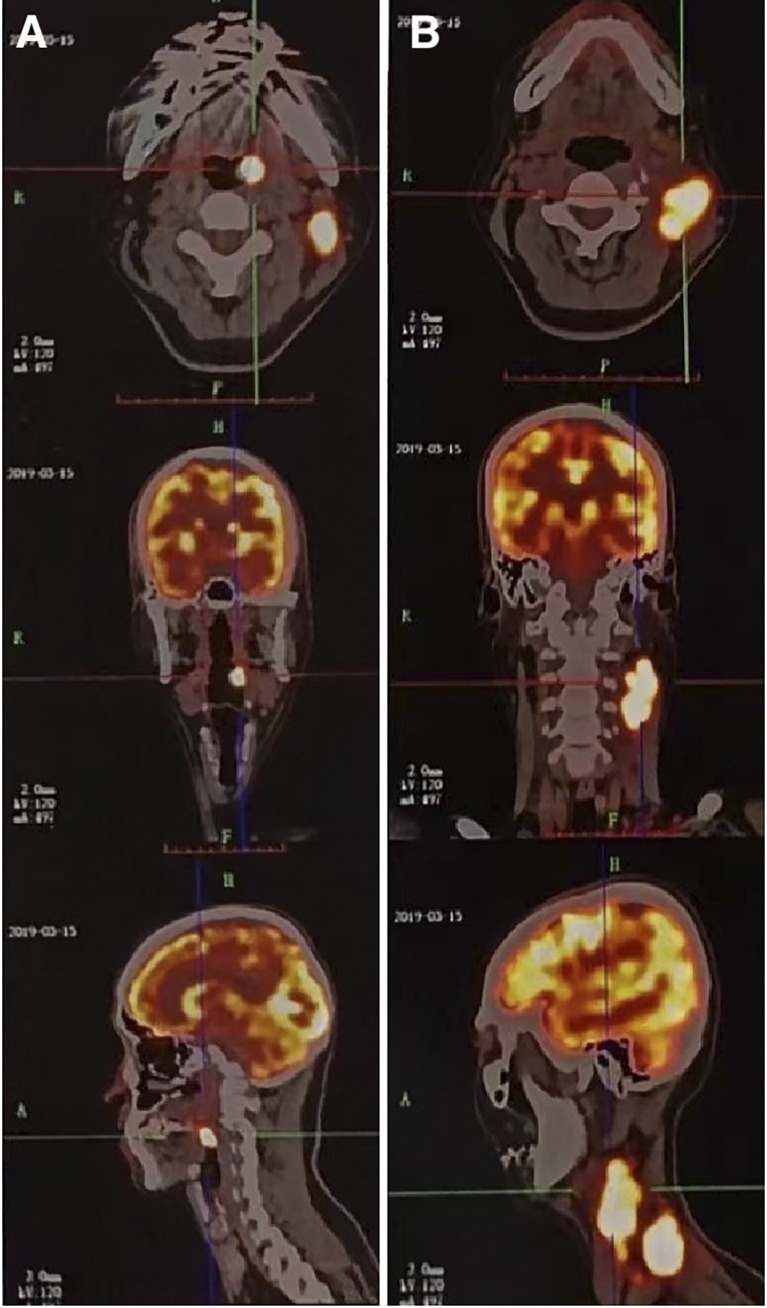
A 51-year-old male patient with tonsillar follicular dendritic cell sarcoma (FDCS). (A) PET/CT scan shows extensive hypermetabolic activity on the left side of the oropharynx (SUVmax 17.7). (B) PET/CT scan shows extensive hypermetabolism in the left neck II, III, IV, and Vb lymph nodes (SUVmax 14.1). CT, computed tomography; PET, positron emission tomography; SUVmax, standardized uptake value maximum.
